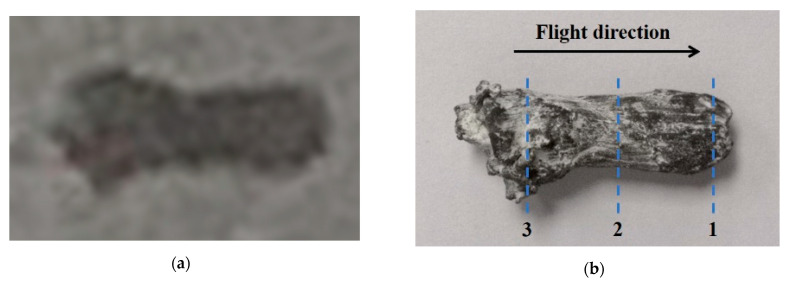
(a) X-ray photograph of EFP forming state at 300 μs and (b) the macro photograph of the recovered projectile. The flight direction indicates the head and tail of the recovered projectile. The observation direction is along the axial direction of the projectile, which is parallel to the flight direction as shown in (b).
